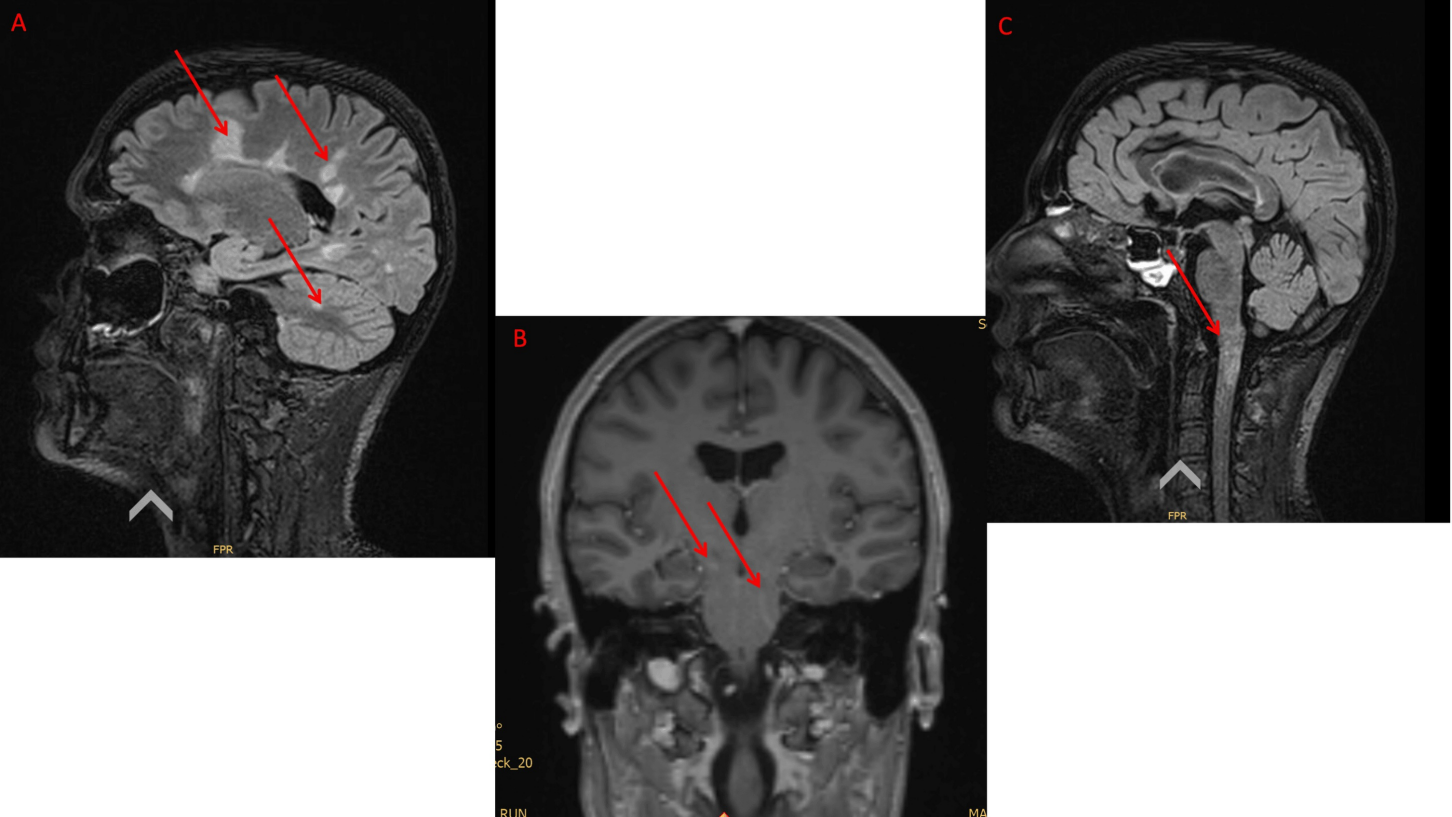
MRI cranium native and with contrast agent on admission day. (A) and (C) T2 flair sagittal: multifocal demyelination in the deep white matter in the supra- and infratentorial region, as well as in the pons and the cerebellar crus on the right. Demyelination is also found at the level of the medulla oblongata and at level C1 (red arrows). (B) T1 sagittal with gadolinium (red arrows).
